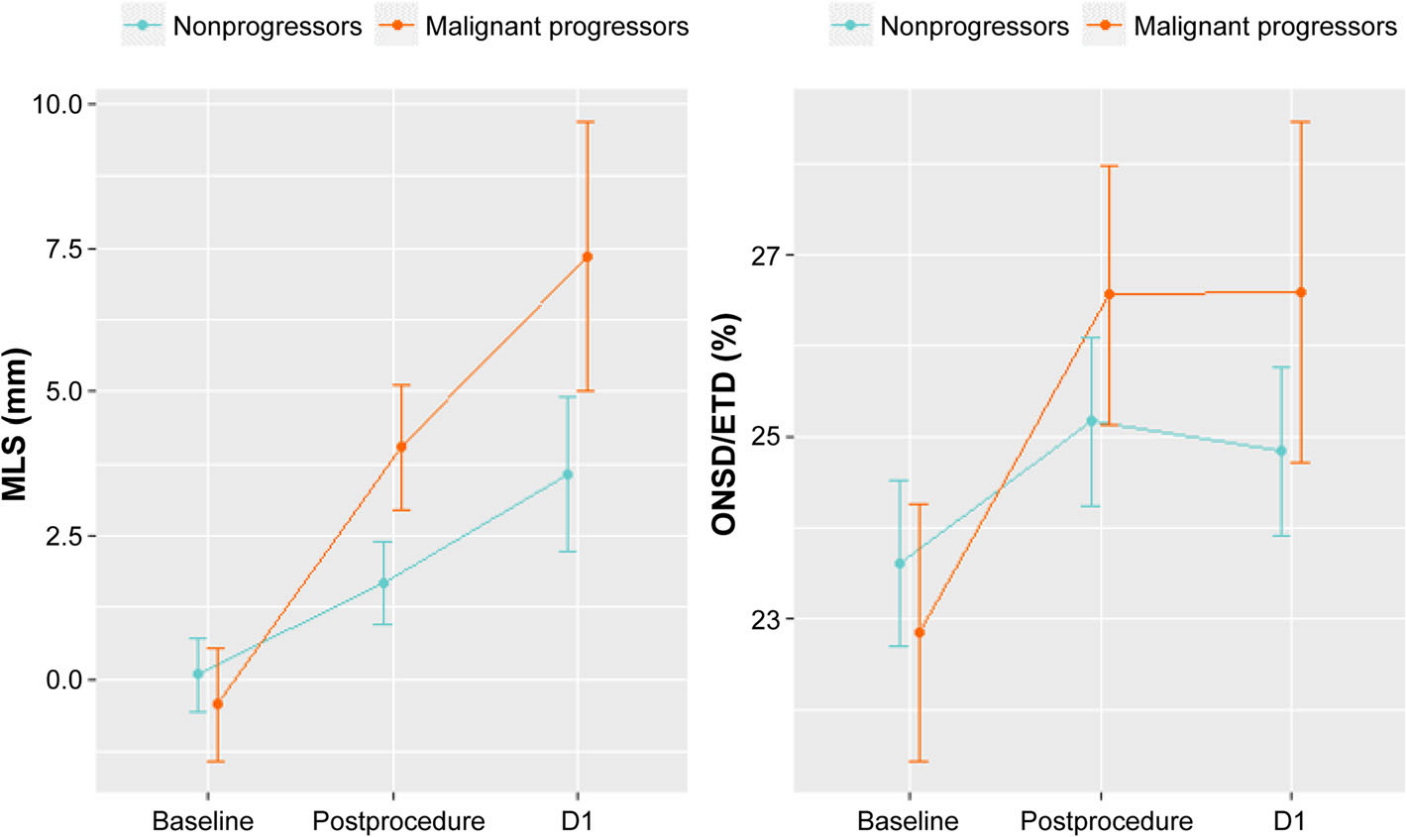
Trajectories of MLS (left panel) and ONSD/ETD (right panel) of follow-up. The time series change in MLS and ONSD/ETD, adjusted for age, sex and DWI volume, are plotted from baseline to D1. The rate of increase of MLS is constantly higher in the malignant progressors throughout the time periods. For ONSD/ETD ratio, it is increased at the postprocedure time point, and this difference is maintained. Due to a slight increase of ONSD/ETD ratio in the postprocedure time point in the nonprogressors, this difference becomes more evident at the D1 timepoint. Error bars represent 95% CIs. MLS, midline shift; ONSD, optic nerve sheath diameter; ETD, eyeball transverse diameter
